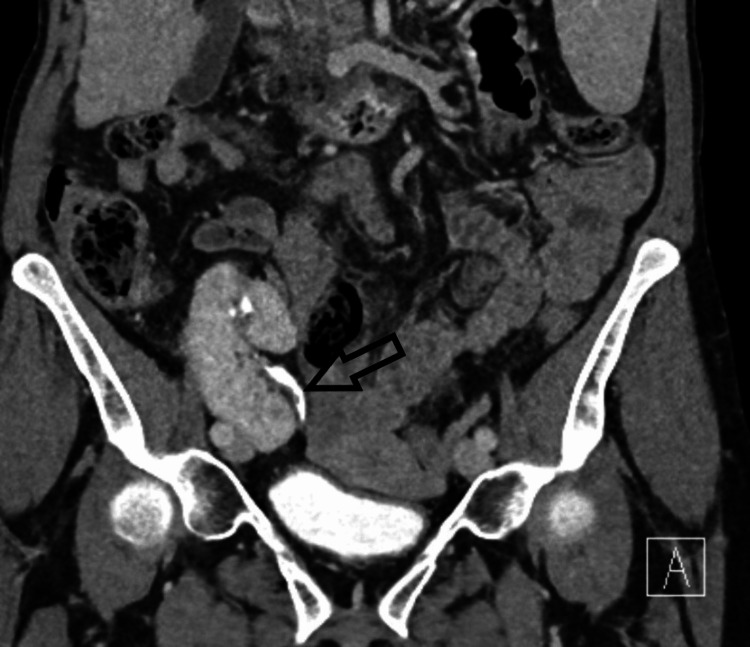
CT urogram of transplanted kidney showing filling defects in the upper ureter
